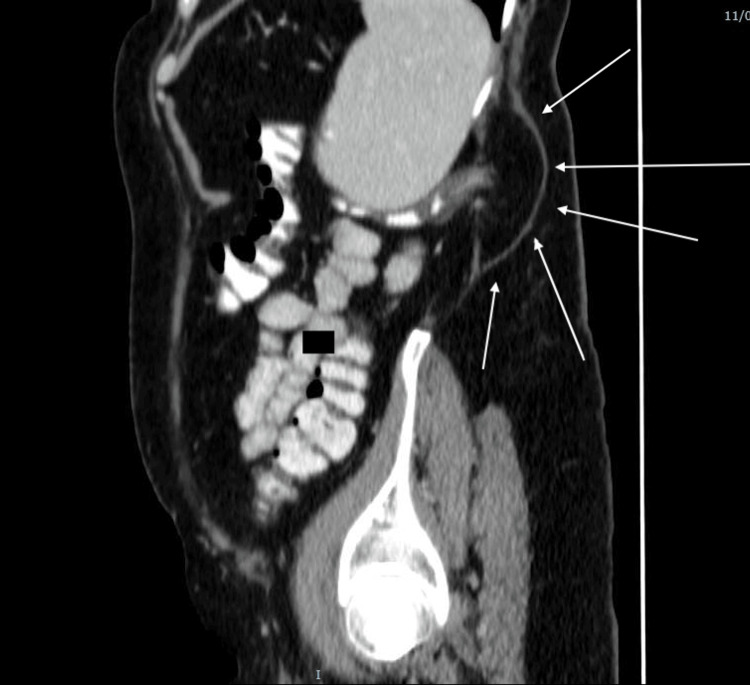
CT sagittal view. The arrows indicate the lumbar hernia defect.
